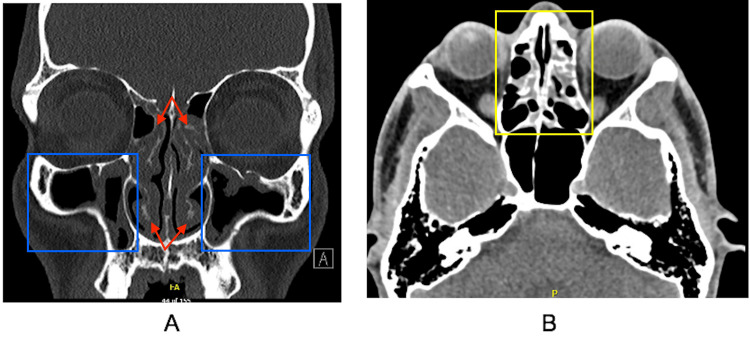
CT scan images of the sinuses Cross-sectional imaging revealing turbinate hypertrophy (red arrows) and polypoid changes of the (A) maxillary sinuses (blue boxes) and (B) ethmoid sinus mucosal thickening (yellow box) CT: computed tomography
